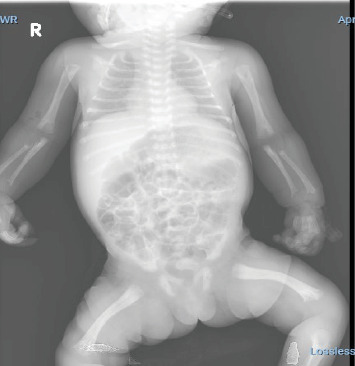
Frontal whole-body radiograph showing skeletal abnormalities. Slender, poorly ossified ribs in the chest, metaphyseal tongues of radiolucency in both upper limbs. Additionally, there is bowing of both femoral bones, absent ossification of the epiphyses of both knees, and metaphyseal tongues of radiolucency involving the distal and proximal tibiae.
